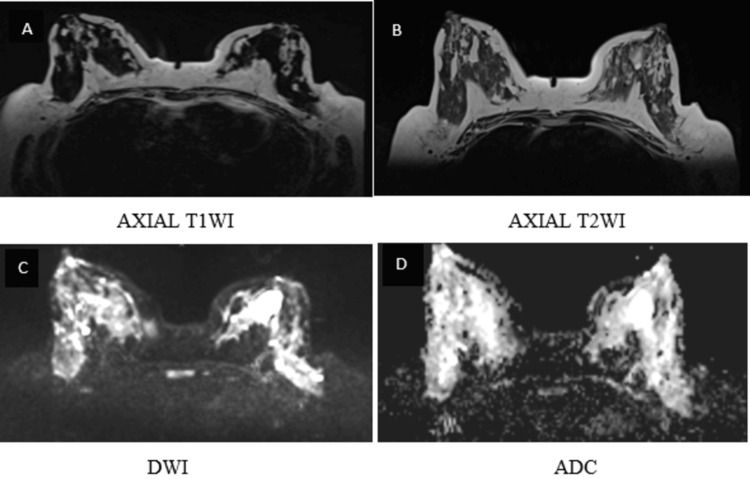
BI-RADS 2 lesion shows a cyst in the left breast, hypointense on T1WI and hyperintense on T2WI (A, B). DWI demonstrates no diffusion restriction (C, D) BI-RADS: Breast Imaging Reporting and Data System; T1WI: T1-weighted image; T2WI: T2-weighted image; DWI: diffusion-weighted imaging; ADC: apparent diffusion coefficient
